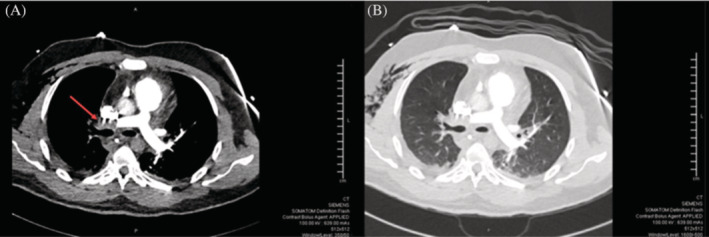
Computed tomography pulmonary angiogram (CTPA) with mediastinal (A) and lung (B) windows showing major right artery pulmonary embolism with reduced vascularity of the right lung
